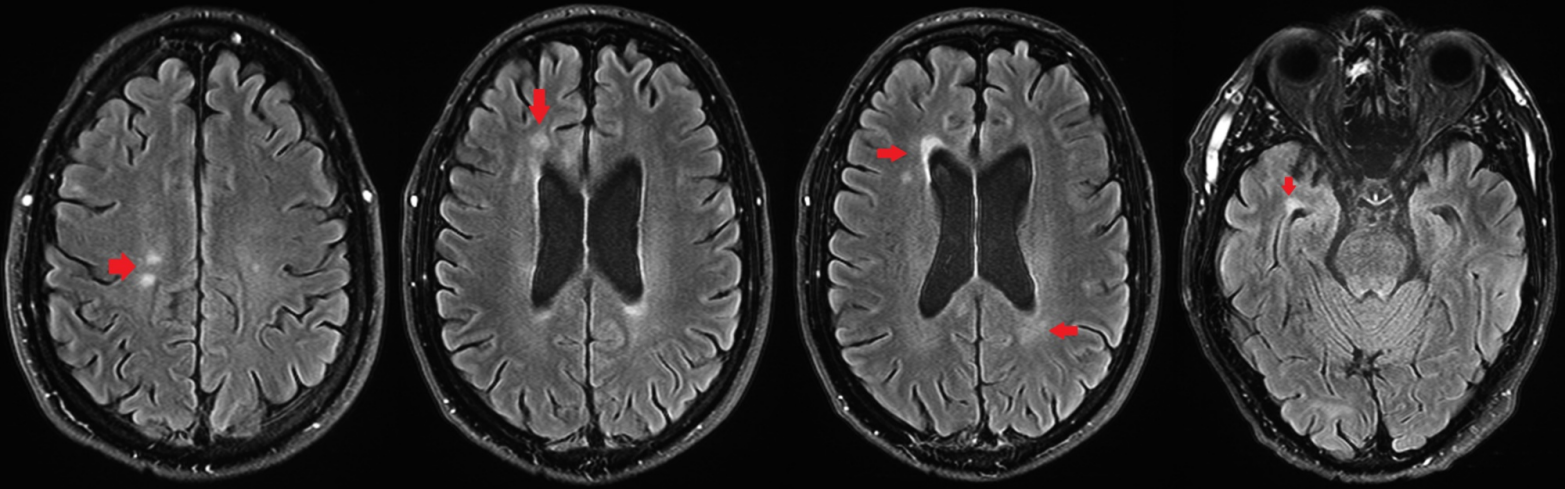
Axial fluid-attenuated inversion recovery (FLAIR) T2-weighted, fat-suppressed brain MRI demonstrating multiple foci of juxta-ventricular signal hyperintensity (arrows).
